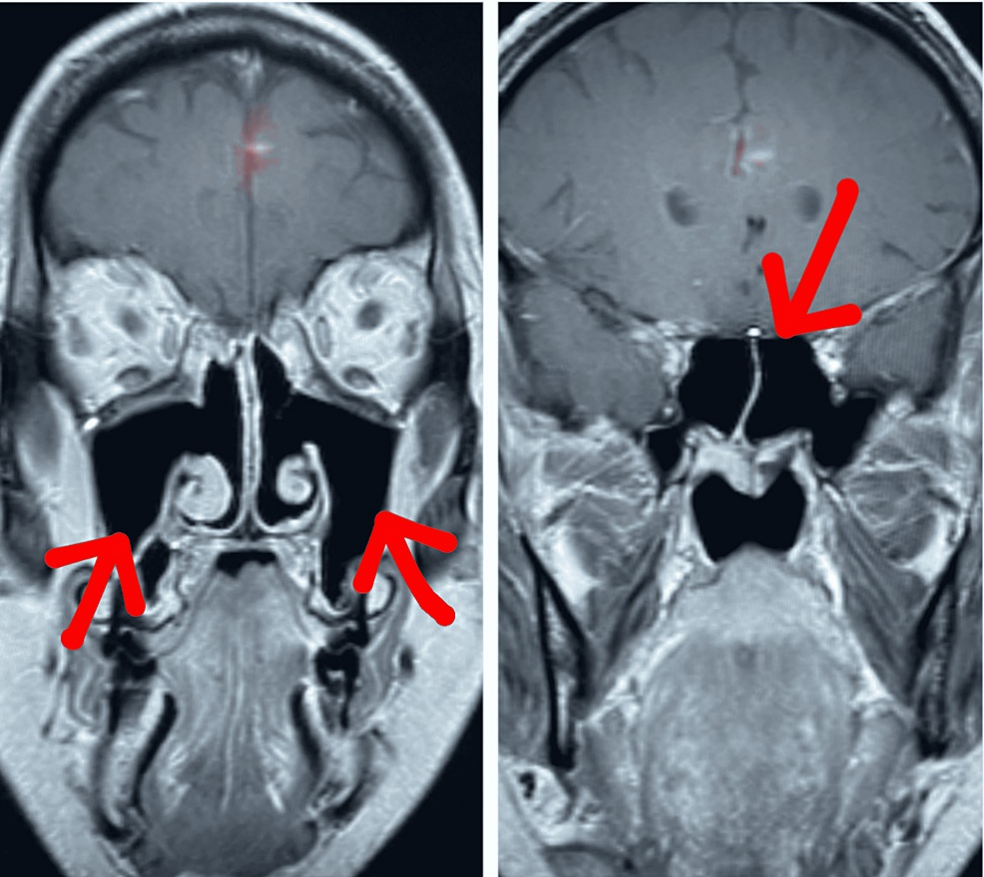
MRI paranasal sinuses post-treatment showing resolution of sinusitis
